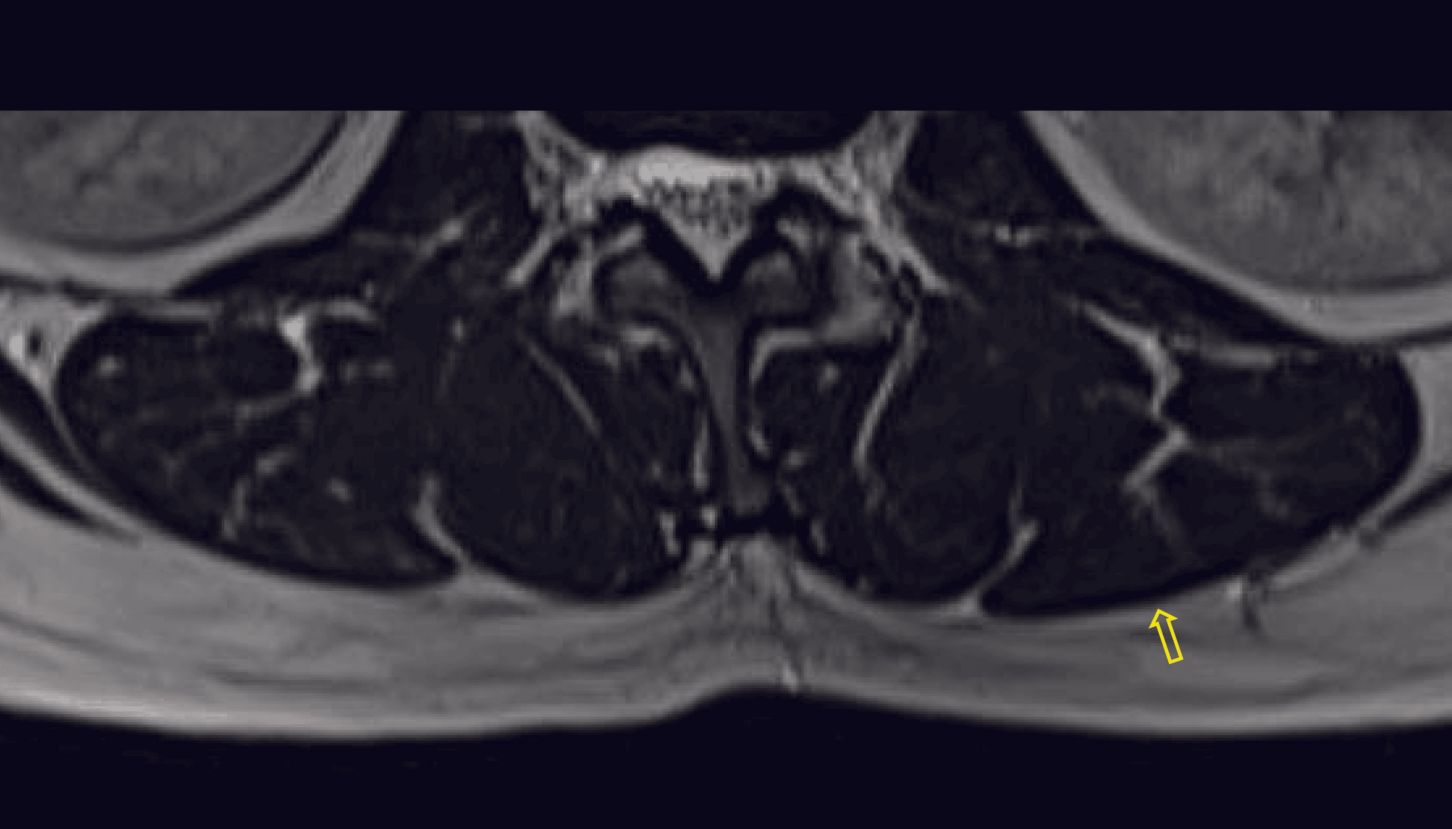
Axial T2-weighted MR image with yellow arrow along the posterior border of paraspinal muscles showing no epimuscular fat deposition, with a score of 0 MR: magnetic resonance
